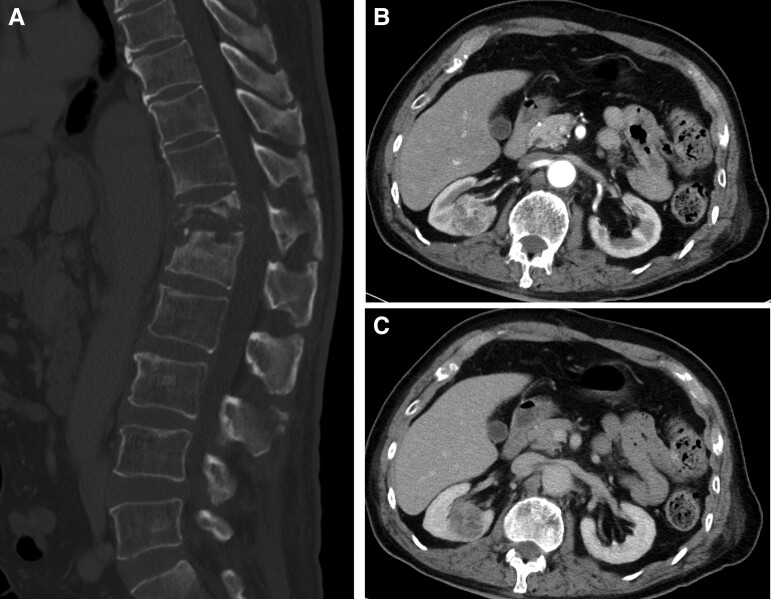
Contrast-enhanced computed tomographic scan showed a pathological fracture and osteolytic changes in the vertebra at the T12–L1 levels. A mass lesion with early enhancement was observed in the right kidney. *A*, Sagittal. *B*, Axial, early arterial phase. *C*, Axial, late phase.
